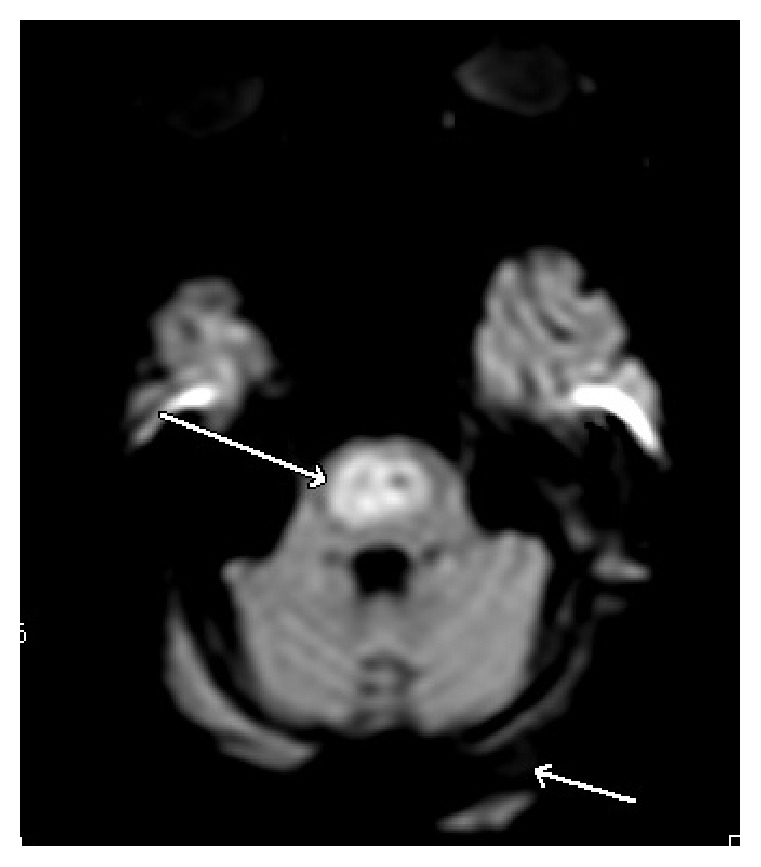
Diffusion weighted MRI image of our patient demonstrating central pontine myelinolysis.
